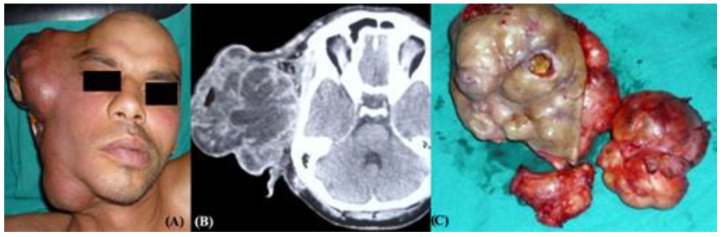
T4-stage CXPA. (A) Clinical image of giant right CXPA; (B) CT scan with contrast media; and (C) complete surgical resection piece.
